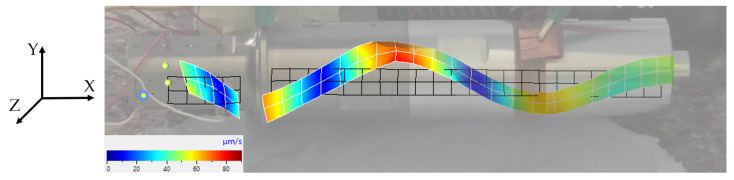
Bending vibration shape of the ultrasonic system.
